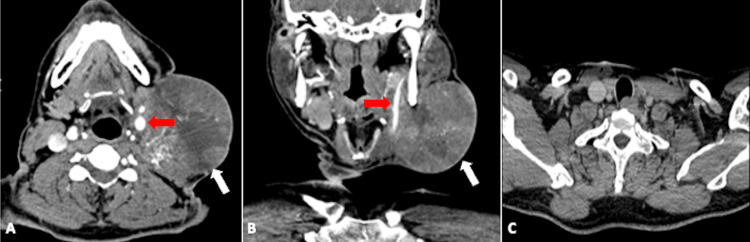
Contrast-enhanced CT scan of the patient with a bleeding nodal mass Contrast-enhanced CT scan axial (A) and coronal (B) images showing a nodal mass on the left side of the neck (white arrows) with encasement of the external carotid artery (red arrows). The nodal mass was closely abutting and displacing the internal carotid artery with an angle of contact of more than 270 degrees (not shown). Normal thyroid tissue is absent in the axial (C) image (postoperative).
